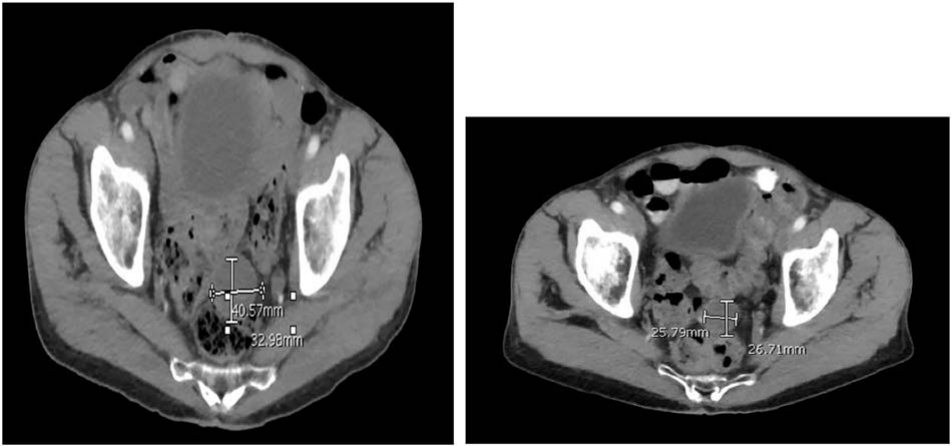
CT abdomen and pelvis at (A) 2- and (B) 4 months follow-up. The pelvic mass measured 4.1 × 3.3 and 2.6 × 2.7 cm, respectively.
